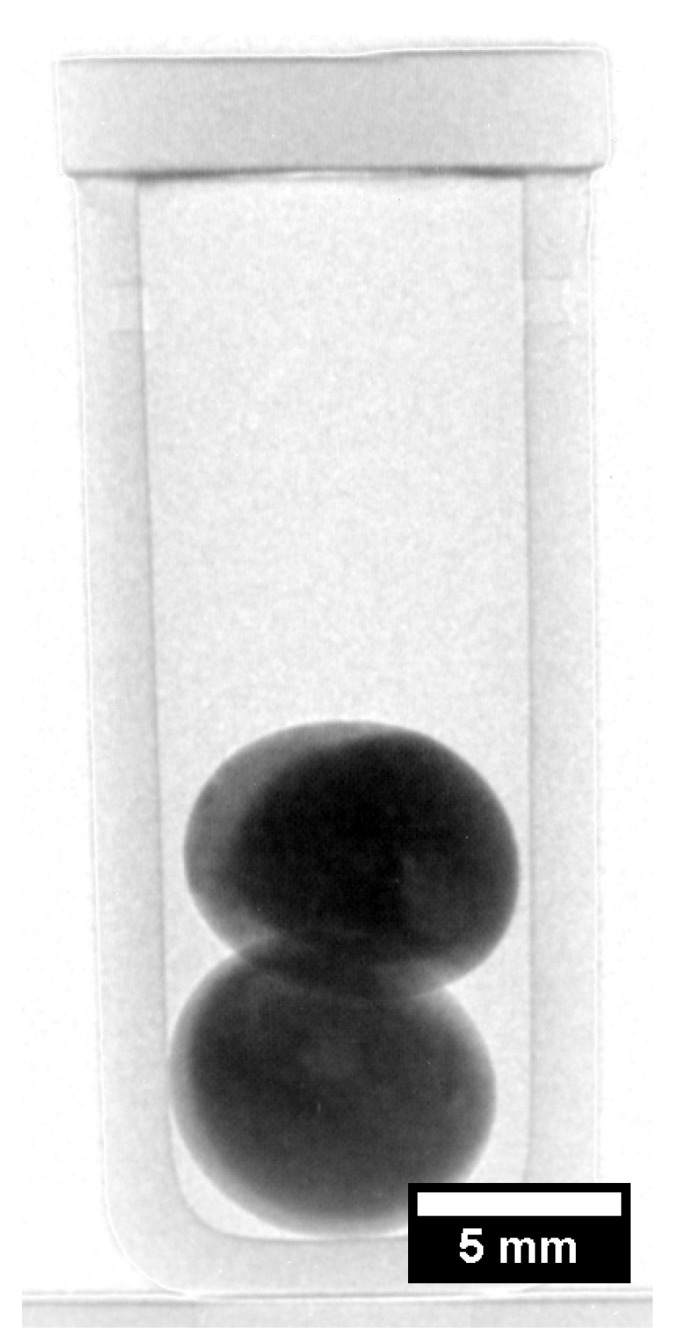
Neutron radiograph of two stacked arc-melted CoCrCu buttons in an alumina crucible with brighter regions corresponding to Cu-rich phases and darker regions corresponding to CoCr-rich phases. Image displayed with permission from the authors in [72].
